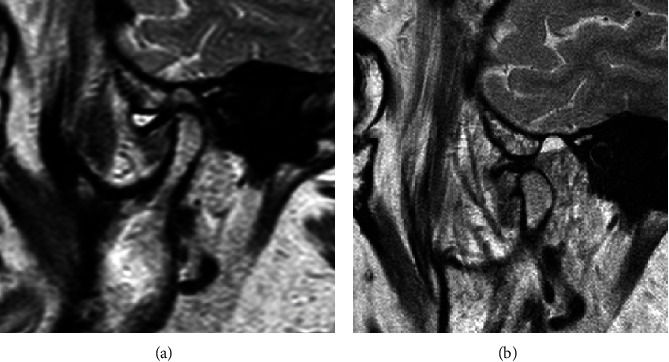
Initial MRI of the left TMJ with the patient's mouth closed (a) and open (b).
